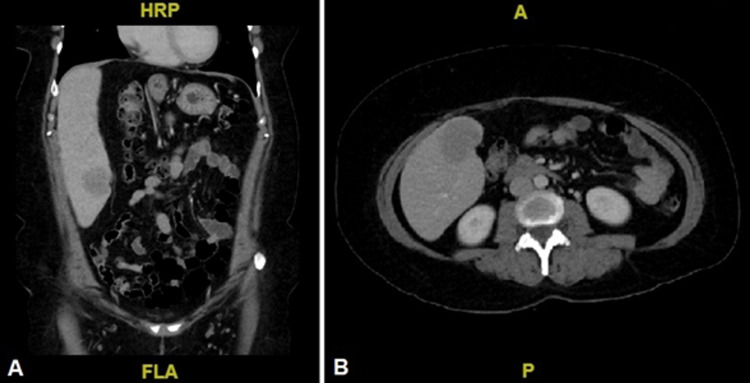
Abdominal CT scan, venous phase. Hypoattenuating hepatic lesion with lobulated margins located in Segment VI, compatible with disease recurrence. A: Coronal view. B: Axial view
